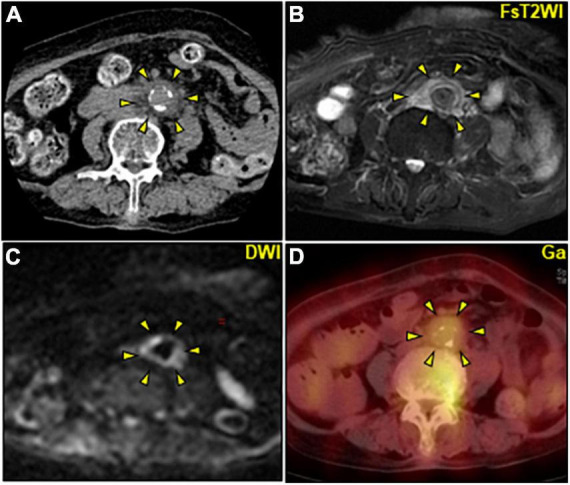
Multimodality imaging of periaortic mass of the abdominal aorta. (A–D) Axial images. (A) Unenhanced CT image showing a non-dilated abdominal aorta with a maximum diameter of 17 mm including mural calcification surrounded by circumferential heterogeneous soft-tissue density (arrowheads). (B,C) Unenhanced abdominal magnetic resonance image. (B) FsT2WI showing significantly high signal intensity and marked thickening of the periaortic tissue of the abdominal aorta (arrowheads) with inhomogeneous diffusion restriction on DWI (C). (D) Ga-single photon emission computed tomography/CT image revealing an active accumulation of Ga (arrowheads) corresponding to the periaortic mass. CT, computed tomography; DWI, diffusion-weighted imaging; FsT2WI, fat-suppressed T2-weighted image; Ga, gallium citrate-67.
